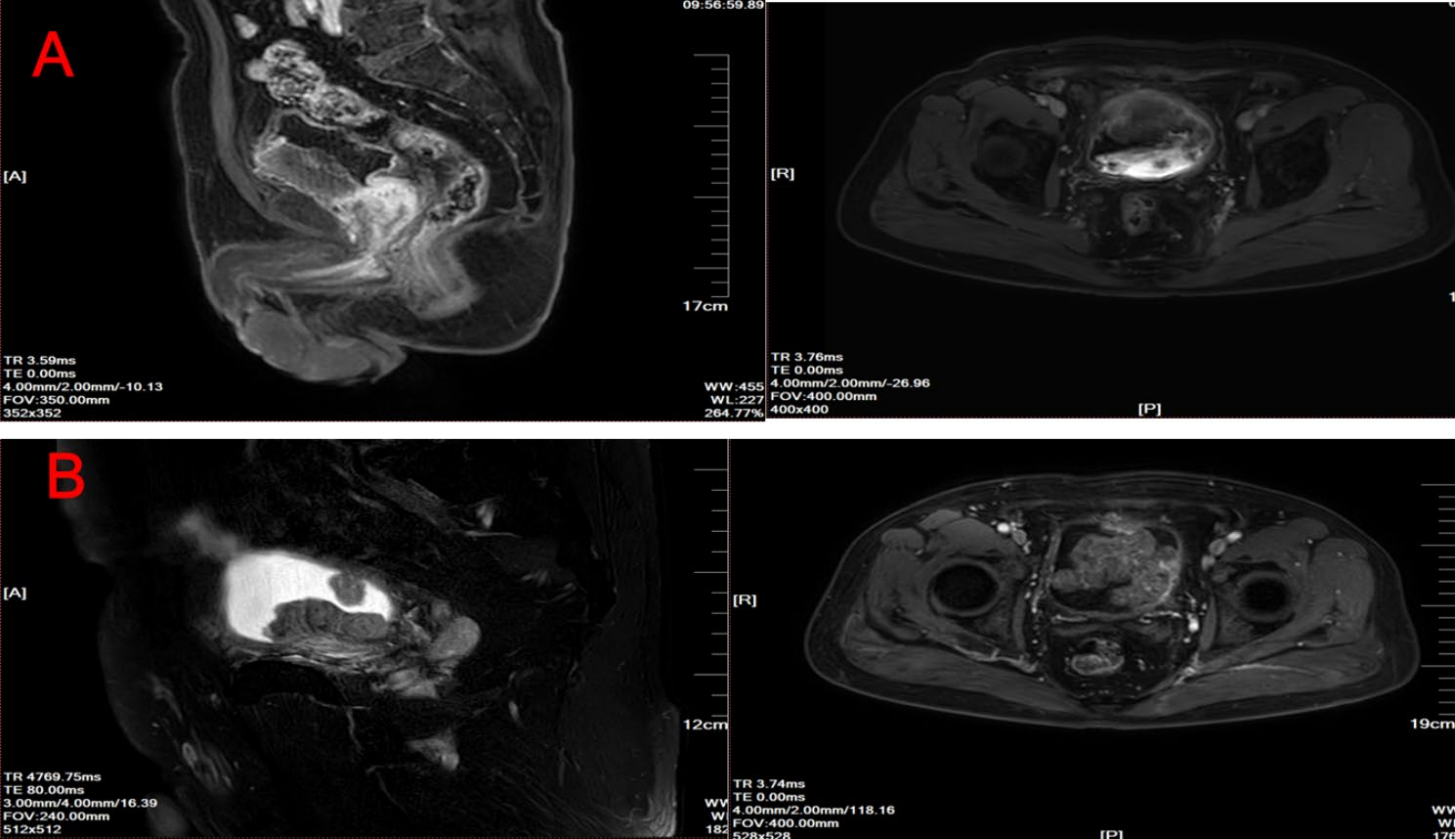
Magnetic resonance imaging of bladder cancer patient. A) Pelvic MRI showing signal abnormalities, the largest which had a size of 5.8*4 cm in the bladder, nodular signal shadow in prostate central zone (sagittal and coronal) in January 2021. B) Pelvic MRI showing irregular and abnormal signal shadow with a size of 8.4*6 cm in the bladder, an abnormal signal in the central gland of the prostate (sagittal and coronal) in May, 2021
